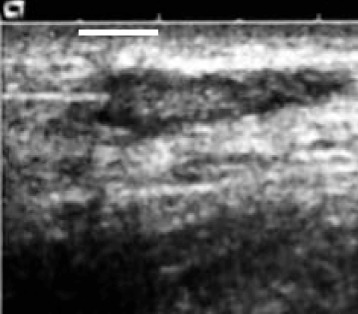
Intralymphatic injection. A sand blasted needle, being inserted into the lymph node from the right was used for better reflection and therefore visibility in the ultrasound. The dark, hypoechoic area represents the paracortex of the lymph node, which is approx. 15 mm long and 5 mm under the skin surface.
